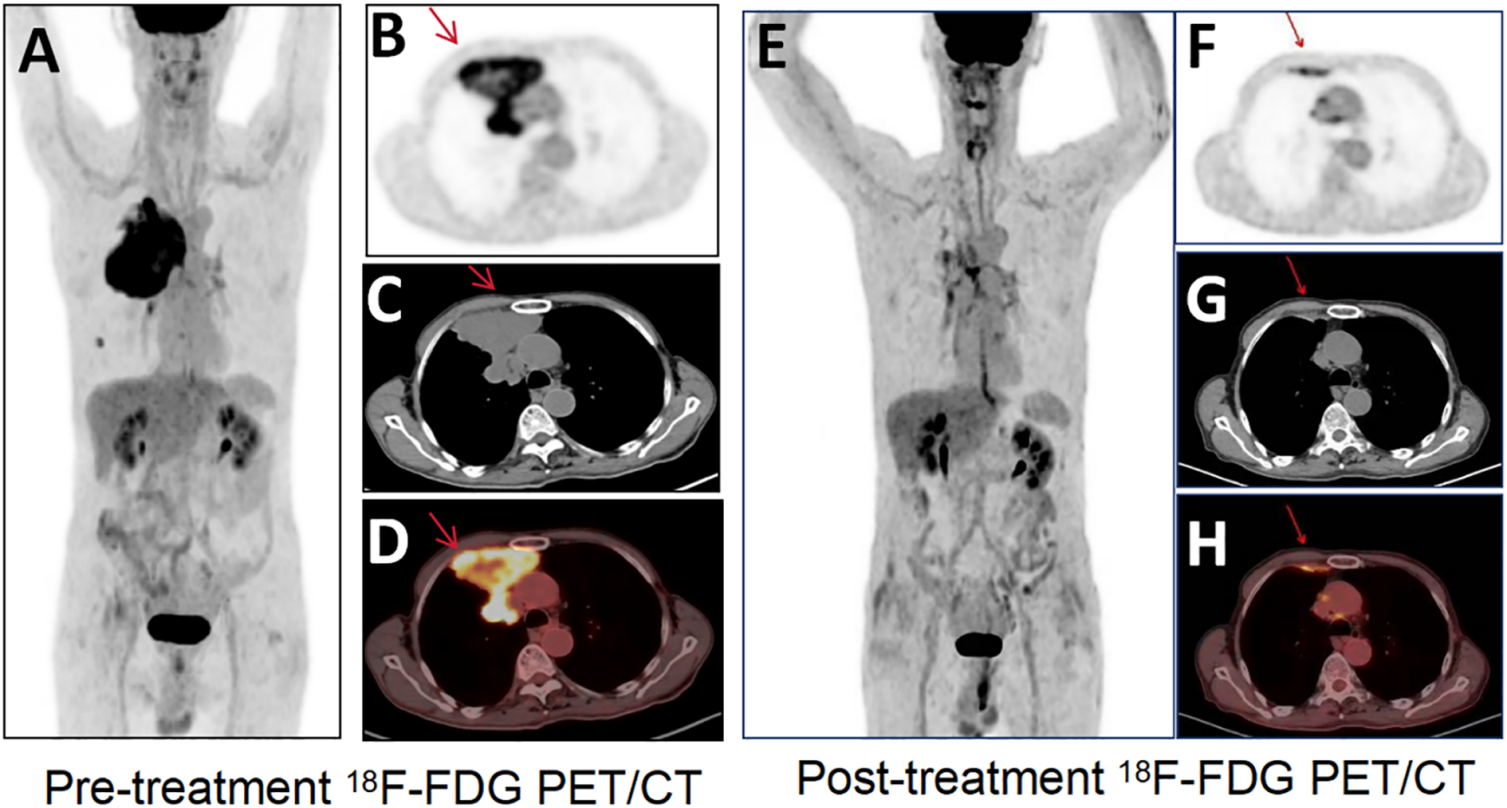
^18^F-FDG PET/CT images. (A) Pre-treatment ^18^F-FDG PET/CT shows high metabolic activity. ^18^F-FDG PET/CT transverse images [(B) PET; (C) CT; (D) fusion] show an irregular soft-tissue-density mass in the anterior-superior region of the right pleura, with a maximal cross-sectional area of approximately (SUVmax 8.2), and a right lung nodule, pleural deposits, and mediastinal lymph nodes. Transverse image (E) Post-treatment ^18^F-FDG PET/CT shows decreased metabolic activity of the primary tumor (SUVmax 2.7) and regression of the nodule/pleural metastasis. Transverse images [(F) PET; (G) CT; (H) fusion] of ^18^F-FDG PET/CT show the corresponding tumors (arrows).
